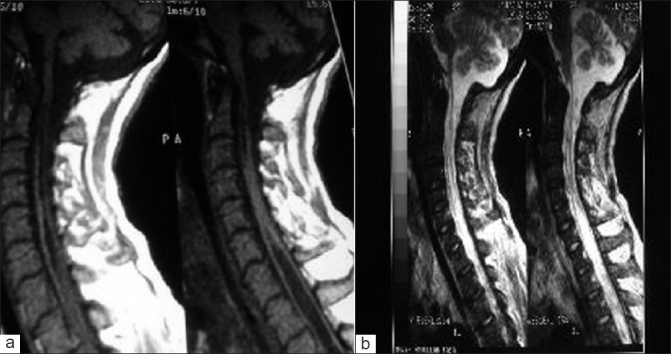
(a,b) MRI of the cervical spine saggital view showing syrinx extending from lower part of medulla to mid-dorsal region
